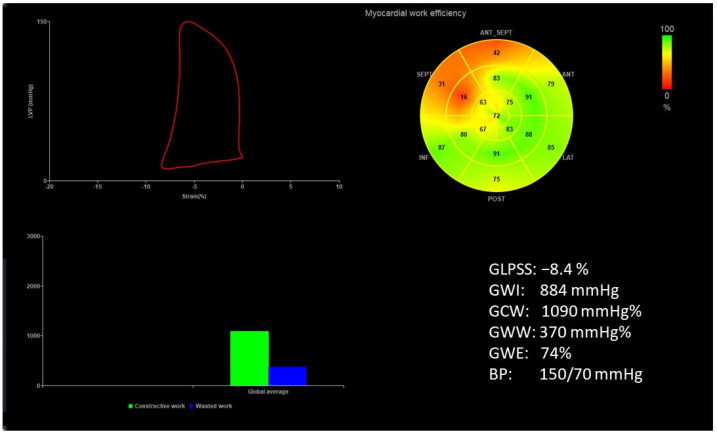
An example of echocardiography of a 61-year-old man with the left bundle branch block and a history of myocardial infarction. A set of individual longitudinal strain curves for all segments shown in different colors, with the average curve shown as a white dotted line, a bull’s eye of segmental longitudinal peak systolic strains and a summary of global longitudinal peak systolic strain (GLPSS) are shown in the top panel. The LV pressure–strain loop with the bull’s eye representation of myocardial work efficiency for each of the segments and a summary of all myocardial work indices are shown in the lower panel. Abbreviations: GLS—global longitudinal peak systolic strain, EF—ejection fraction, GWI—global myocardial work index, GCW—global constructive work, GWW—global wasted work, GWE—global work efficiency, BP—blood pressure.
